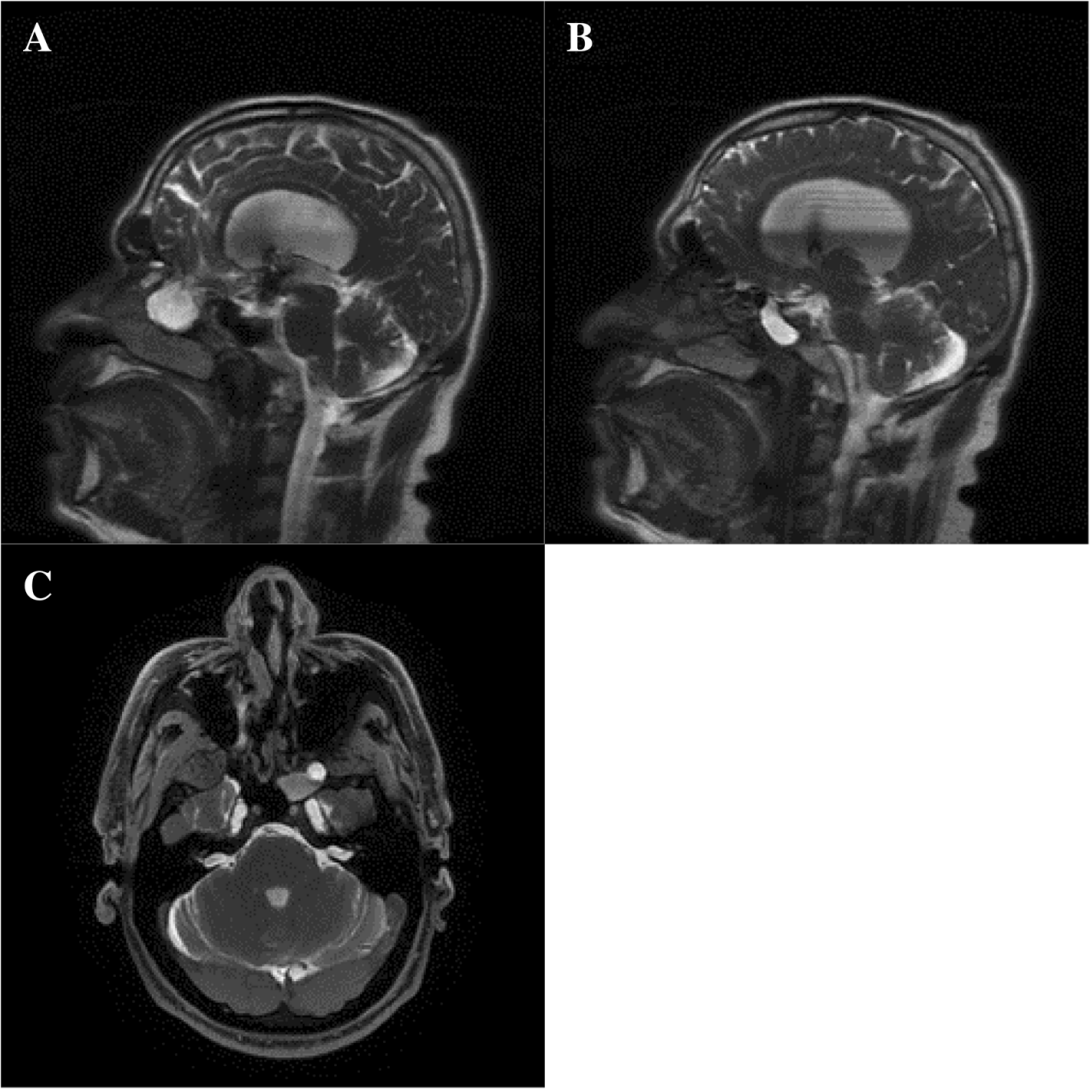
Magnetic resonance imaging cisternography, preoperative images. a and b Sagittal magnetic resonance imaging cisternography images showing right anterior ethmoidal (a) and left sphenoidal (b) meningoceles. c Axial magnetic resonance imaging cisternography image showing left sphenoidal meningocele
